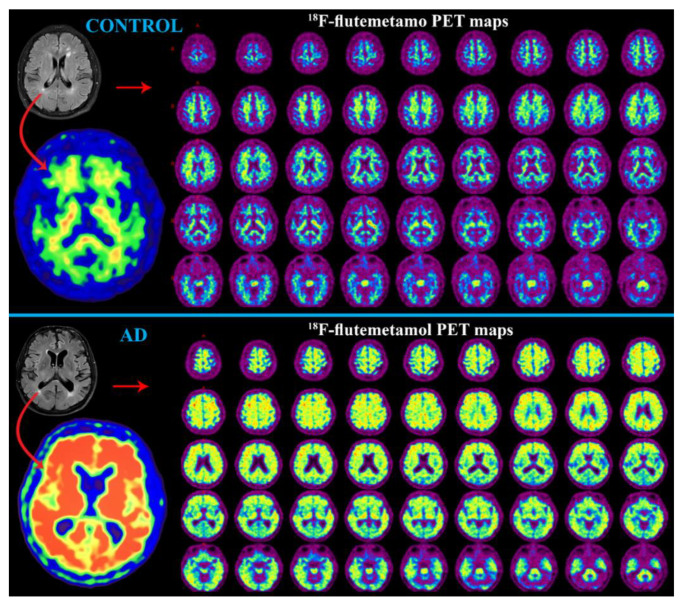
Example of ^18^F-flutemetamol (Vizamyl) PET neuroimaging of a control subject (73-year-old male) and AD patient (72-year-old male). Note the visualization of subtle cortical atrophy with enlarged ventricles (T_1_-weighted MRI at 3 Tesla MR-scanner) and increased retention of the Vizamyl radiotracer demonstrating the presence of Aβ deposition in the AD brain tissue, compared with that of the control subject.
